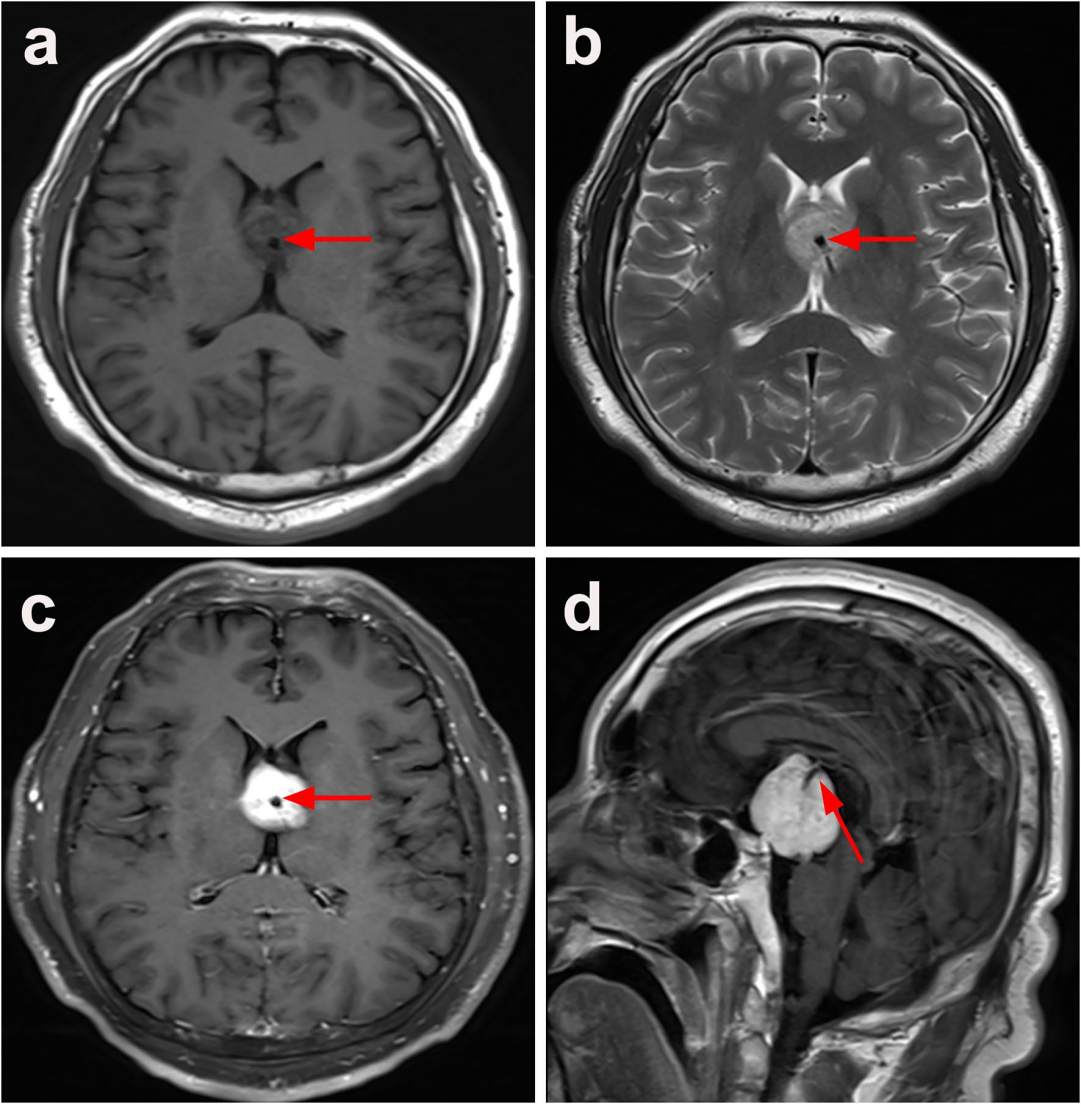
Brain magnetic resonance imaging showing flow void within the tumor. The mass mainly shows isointensity on axial T1WI (a) and T2WI (b); Postcontrast axial (c) and saggital (d) T1WI show a suprasellar lesion with a well-defined margins and obvious homogeneous enhancement. Note that within the mass there is a flow void (arrows in a–d) demonstrating the strong arterial supply of a highly vascularized tumor
